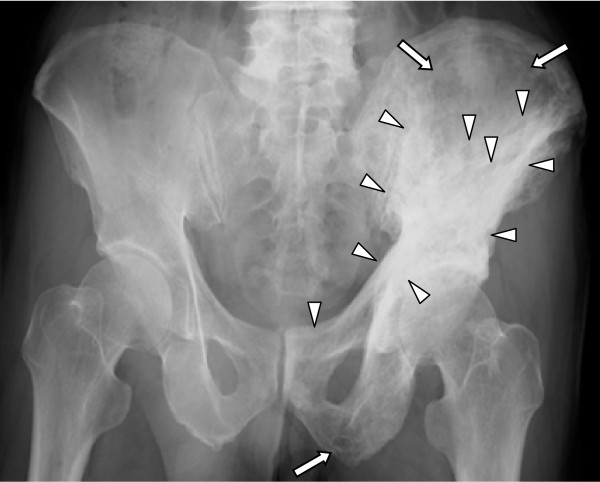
Radiography of patient 1 showed pagetic changes as osteolytic (white arrow) and sclerotic (white arrow head) changes in the left acetabulum, half of the ilium, and the pubic bone.
